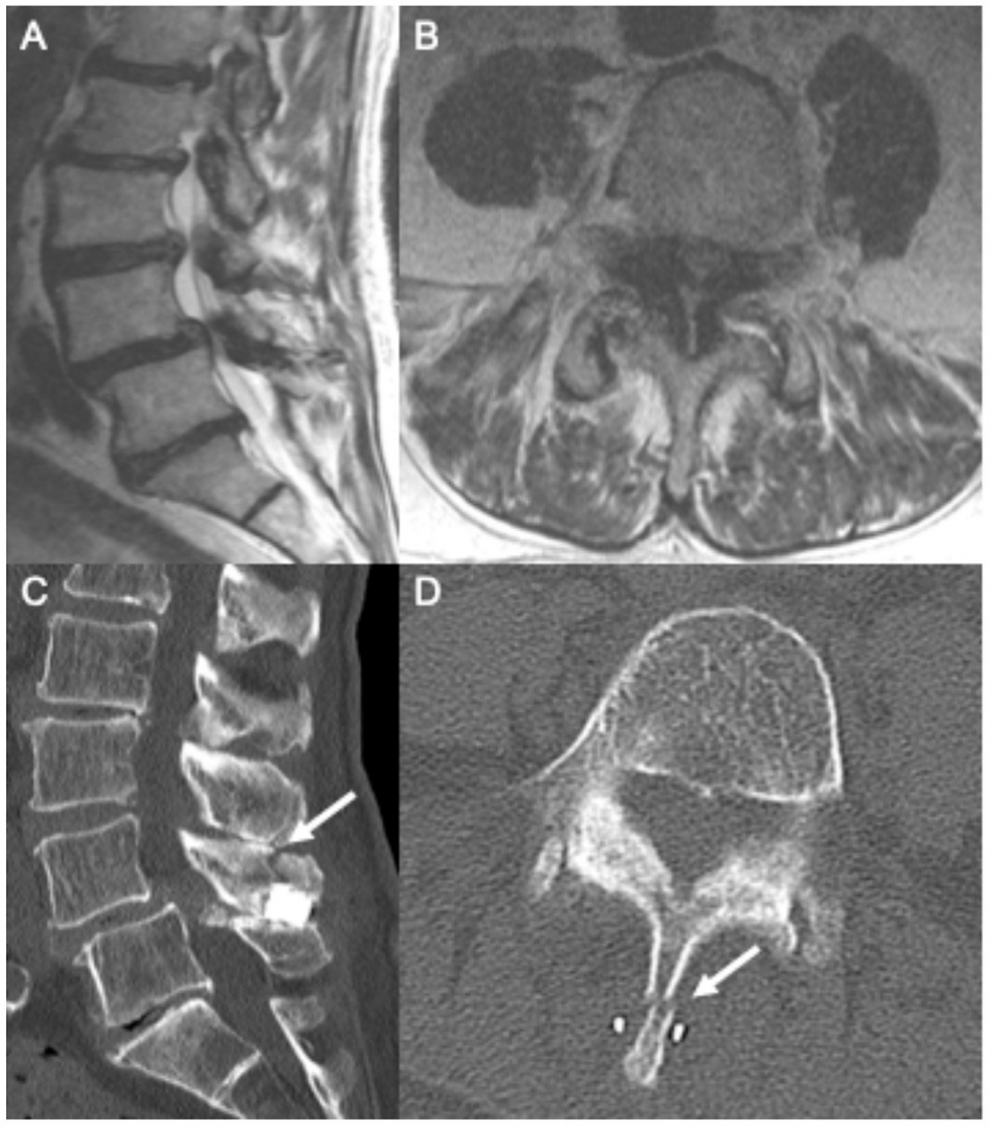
Pre-operative sagittal (A) and axial (B) T2 MRI showing grade 1 spondylolisthesis and L4/5 severe central canal stenosis. Following interspinous spacer placement, sagittal (C) and axial (D) CT imaging showing L4 spinous process fracture.
